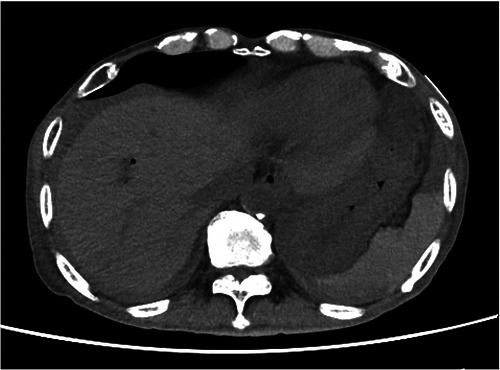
The hematoma around the gastrosplenic ligament showed improvement on follow‐up computed tomography (CT) obtained 2 days after endoscopic retrograde cholangiopancreatography (ERCP).
